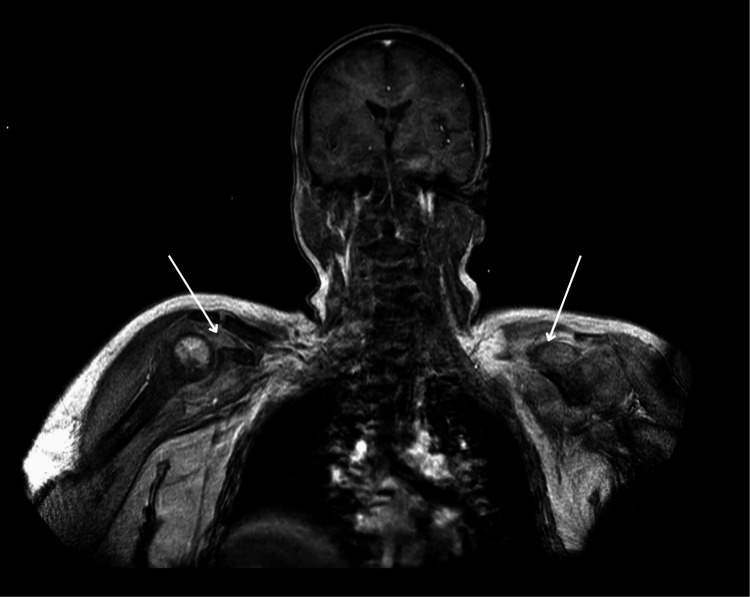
MRI of the upper thorax and shoulder region with contrast (T2 sequence, coronary view) showing hyper-intensity of the shoulder muscles surrounding the shoulder girdle, indicating active myositis.
